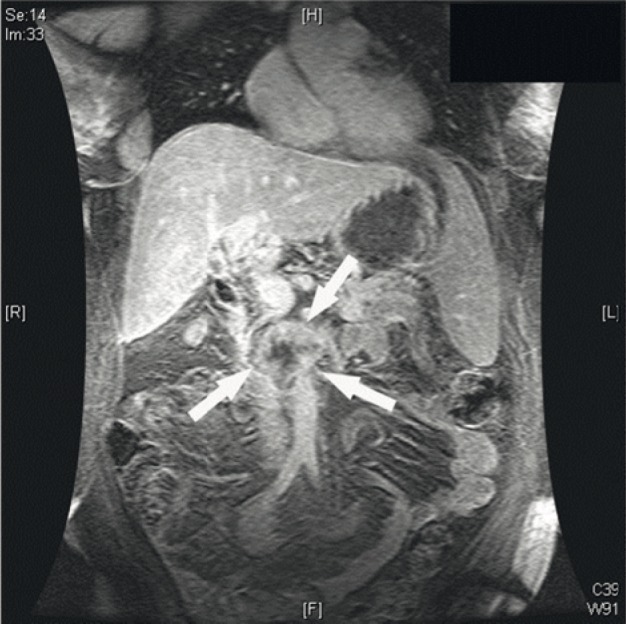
Magnetic Resonance Imaging (MRI) After Liver Transplantation Showing Enlarged Hilar and Paraaortic Lymph Nodes, Which Were Proven to be a Metastatic Cholangiocarcinoma
